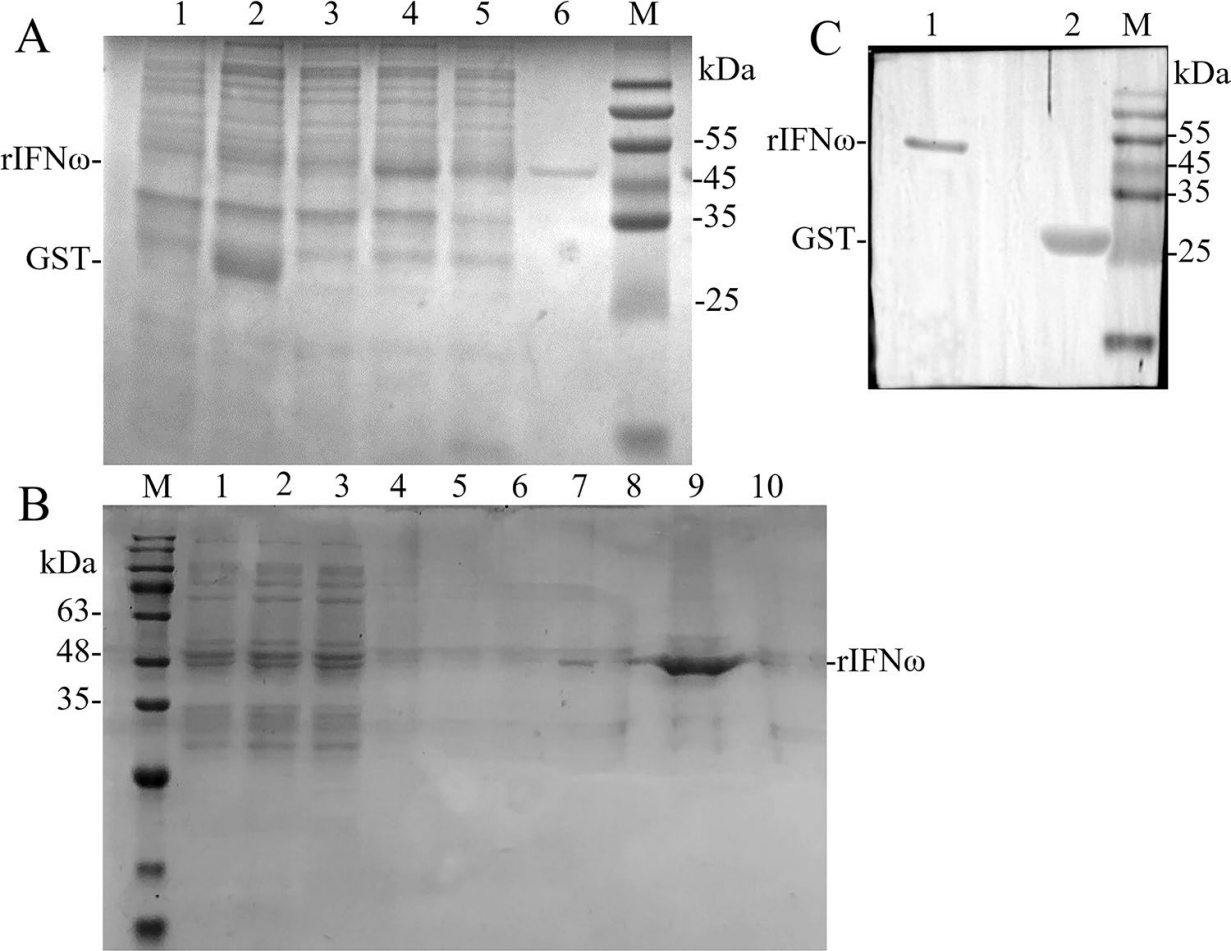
Expression, purification and confirmation of rIFNω proteins from *E. coli* BL21. (A) SDS-PAGE results of preliminary induction of rIFNω expression. Note: M, protein molecular weight standard (15–120 kDa); 1, uninduced bacteria with untreated pGEX-6P-1; 2, induced bacteria with untreated pGEX-6P-1; 3, uninduced rBL21; 4, induced rBL21; 5, the supernatant of induced rBL21 after ultrasonic crushing; 6, precipitation of induced rBL21 after ultrasonic crushing. (B) Purification result of rIFNω. Note: M, protein molecular weight standard (11–180 kDa); 1–3, flow through liquid; 4–5, rinse liquid; 6–8, eluent; 9, ultrafiltration concentrate rIFNω; 10, filtrate. (C) Western blot confirmation. Note: 1, purified rIFNω; 2, induced bacteria with untreated pGEX-6P-1. M, Protein molecular weight standard (15–120 kDa).
